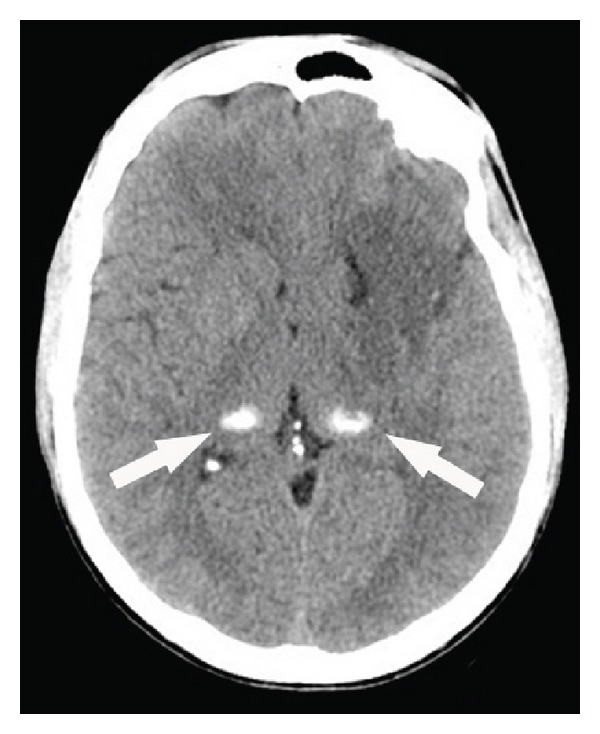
A patient with atypical Werner syndrome (i.e., a progeroid syndrome with Werner syndrome phenotype but without typical* RECQL2* mutation) due to a* LMNA* mutation showing bilateral thalamic calcifications on CT scan.
